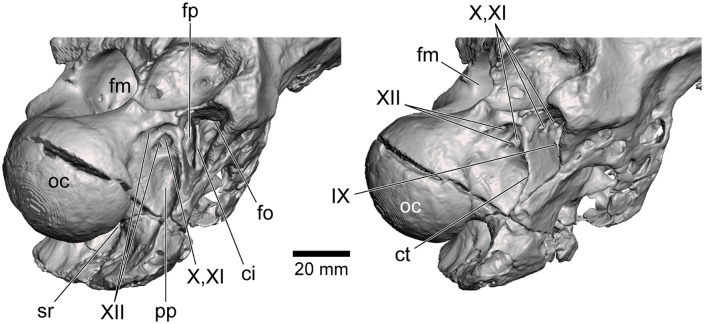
Exoccipital region in CT-based 3D rendered model of NRRU-F01020035 in posterolateral (left) and lateral slightly-posterior (right) views. Abbreviations: ci, crista interfenestralis; ct, crista tuberalis (metotic strut); fm, foramen magnum; fo, fenestra ovalis; fp, fenestra pseudorotunda; oc, occipital condyle; pp, paracondylar pneumatopore; sr, subcondylar recess; IX–XII, exits of respective cranial nerves.
